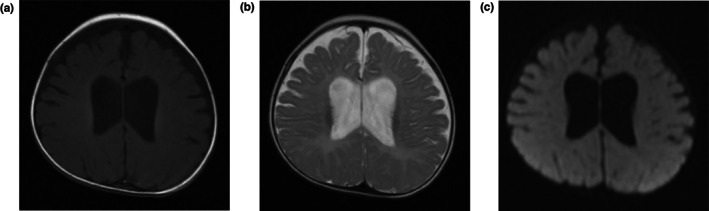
Brain MRI of the patient showed cerebral dysplasia and bilateral lateral cerebral ventriculomegaly. (a) T1W; (b) T2W; (c) DW.
